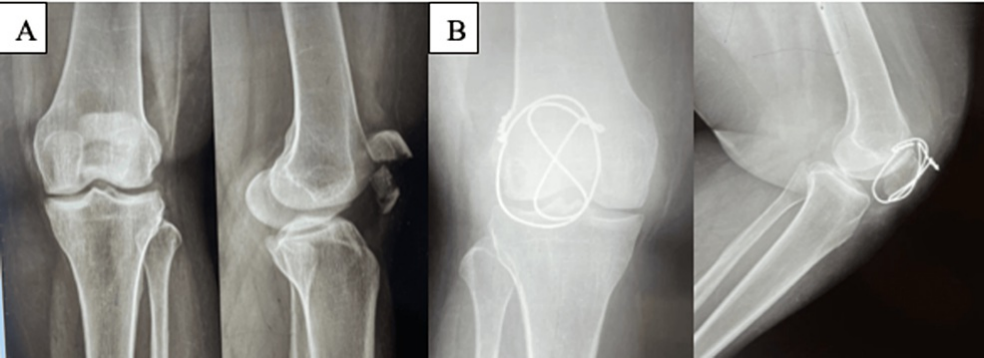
(A) Preoperative X-rays. (B) Follow-up X-rays showing the complete union of patella fracture at 16 weeks
